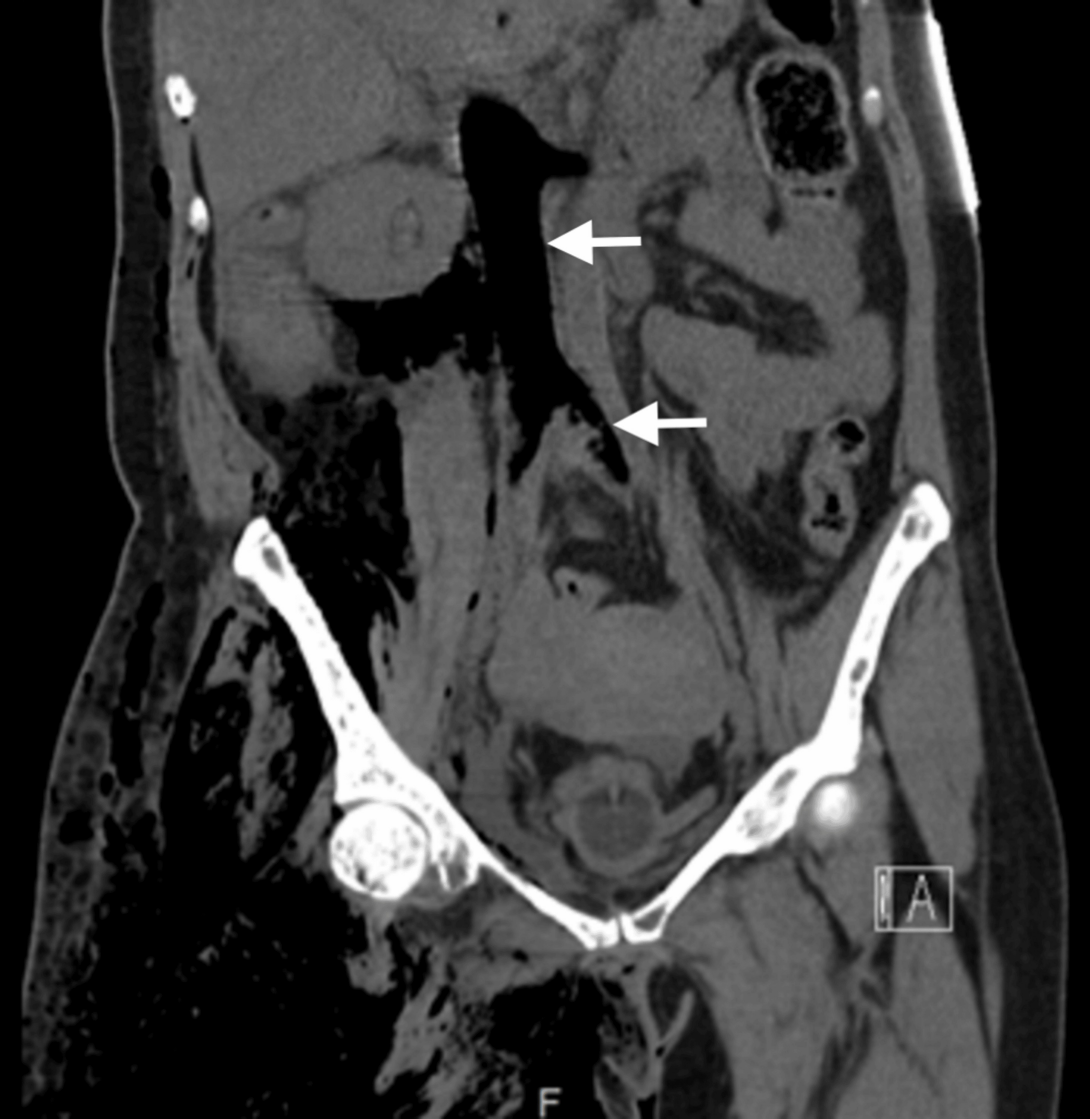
Coronal CT scan of the abdomen and pelvis showing extensive gas dissection from the right thigh into the retroperitoneum, with intravascular gas in the iliac venous system and inferior vena cava (white arrows).
